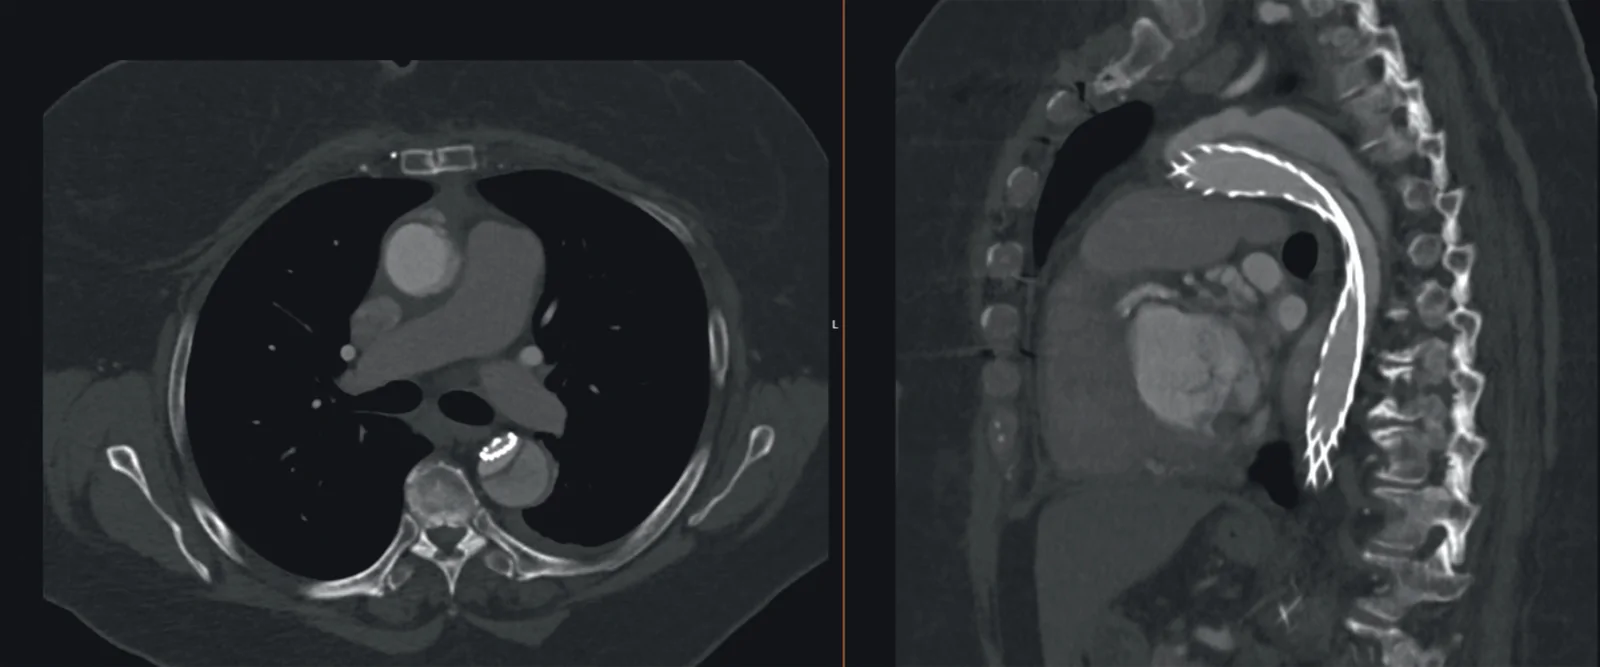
Axial and sagittal CT images demonstrating central stent
